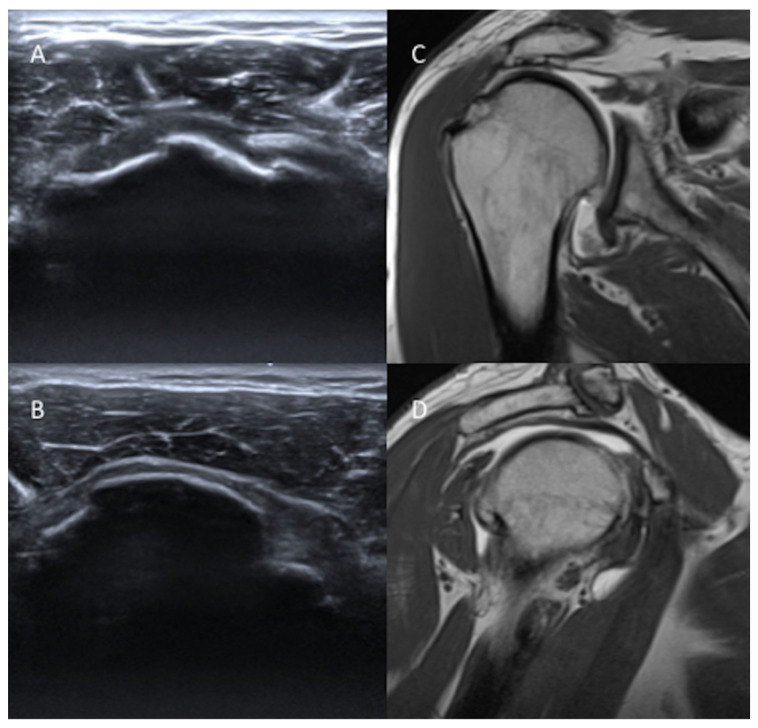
US images on the long axis (A) and short axis (B), coronal T1-weighted (C), and sagittal T1-weighted (D) MRA images of a 57-year-old male patient with complete supraspinatus tendon rupture.
